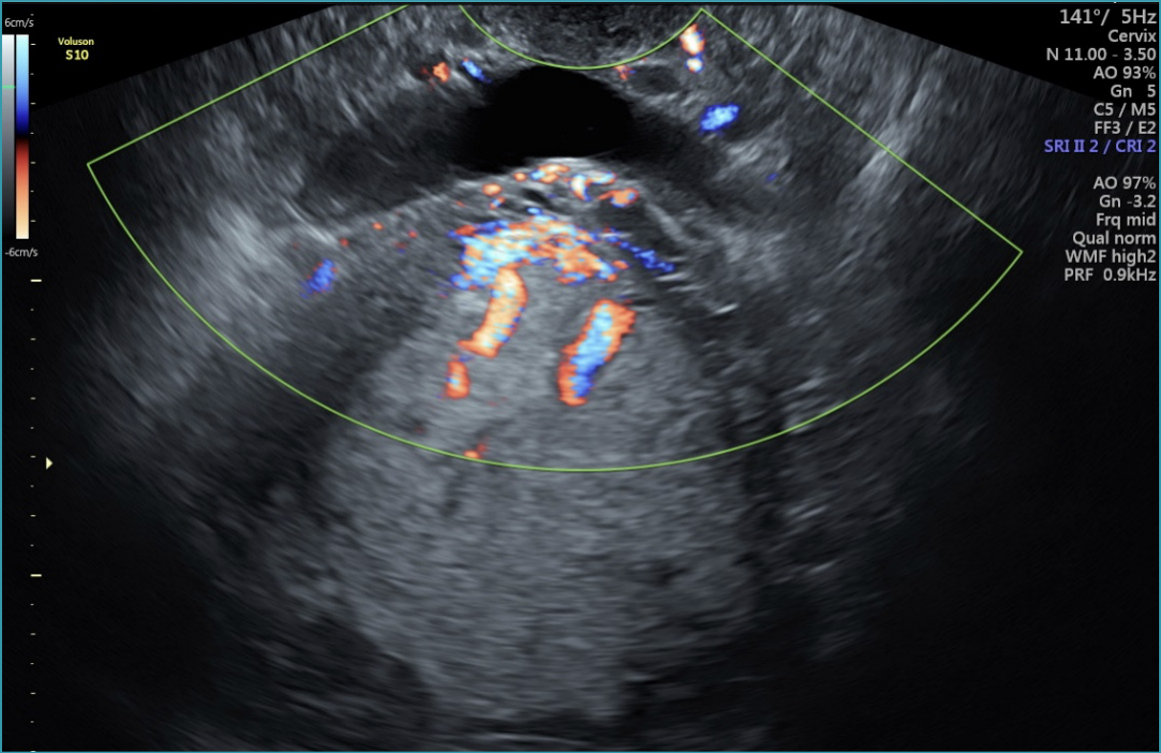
Cross-section of the uterovesical surface performed transvaginally with B-mode associated with color Doppler showing bridging vessels between placenta and bladder
